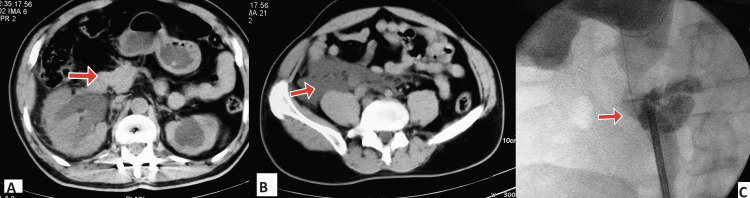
Computed tomography images with axial cuts showing (A) right hydronephrosis with (B) urinary extravasation. (C) Retrograde pyelogram (antero-posterior view) showing extravasation of contrast, as marked by the arrows.
